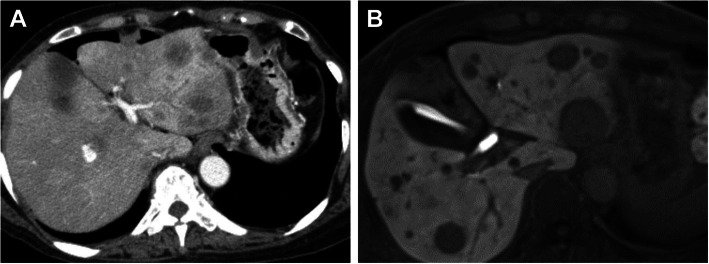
a Computed tomography showed multiple liver metastases in the both liver lobes. b Magnetic resonance imaging
